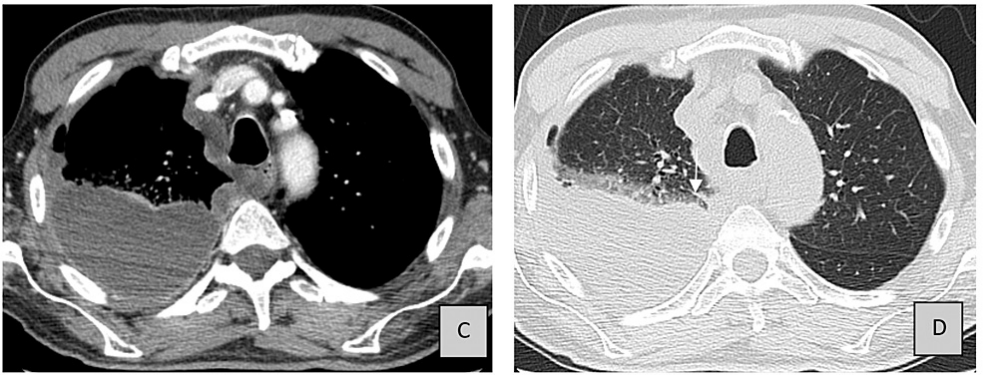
CT of the thorax. A contrast-enhanced axial CT image of the thorax, transverse cut (panels C and D), presenting a large pleural effusion and thickening of soft tissues, involving the anterior and superior right hemithorax, insinuating into the mediastinum, with involvement of the pleura mediastinal on its right side.
